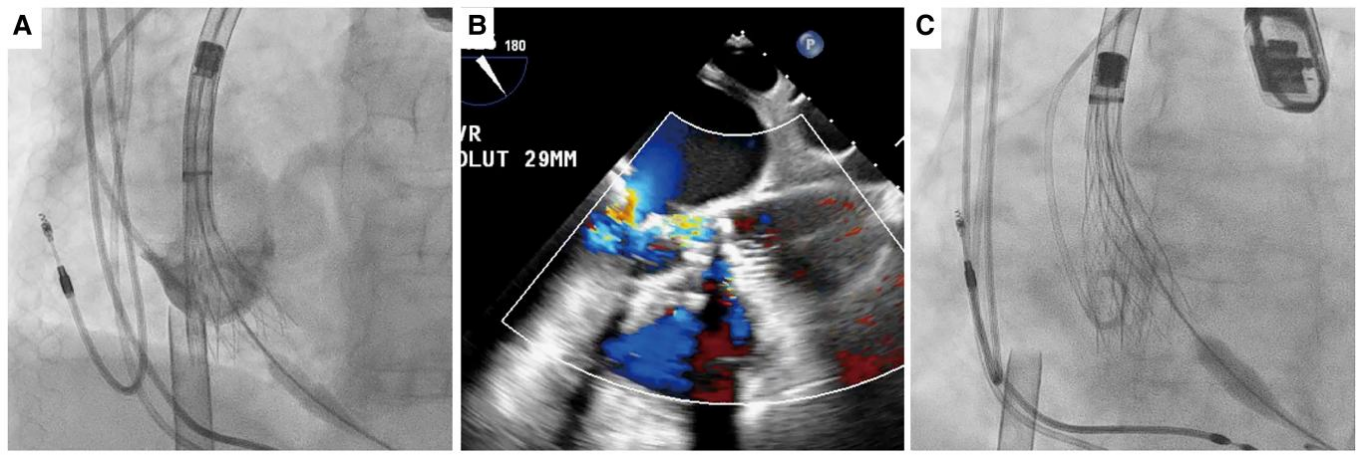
(*A*) The first 29 mm valve resulted in massive paravalvular leakage due to a lack of coaxiality. (*B*) Transoesophageal echocardiography image of paravalvular leakage with the first 29 mm valve. (*C*) The next 34 mm valve caused stent-frame infolding.
